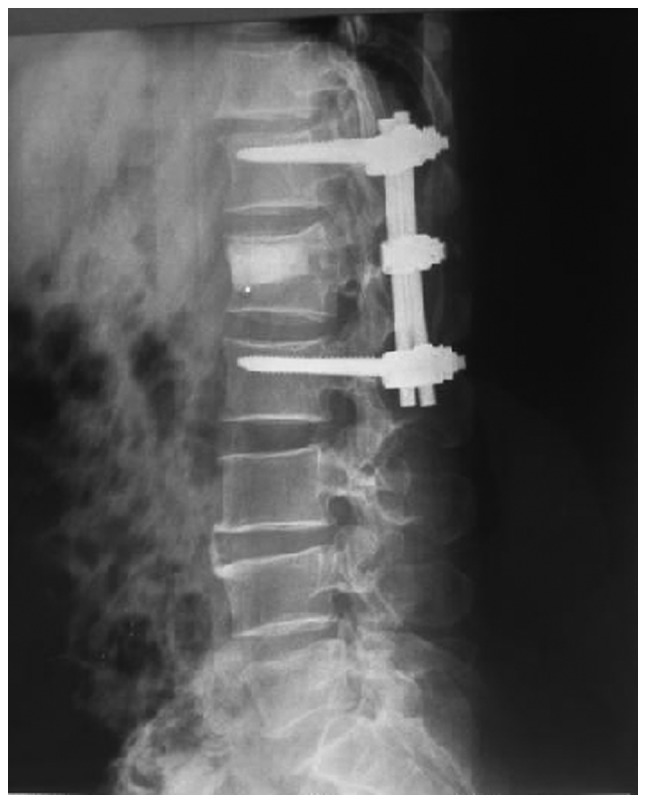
Postoperative X-ray film of lumbar vertebra of a 45-year-old male.
